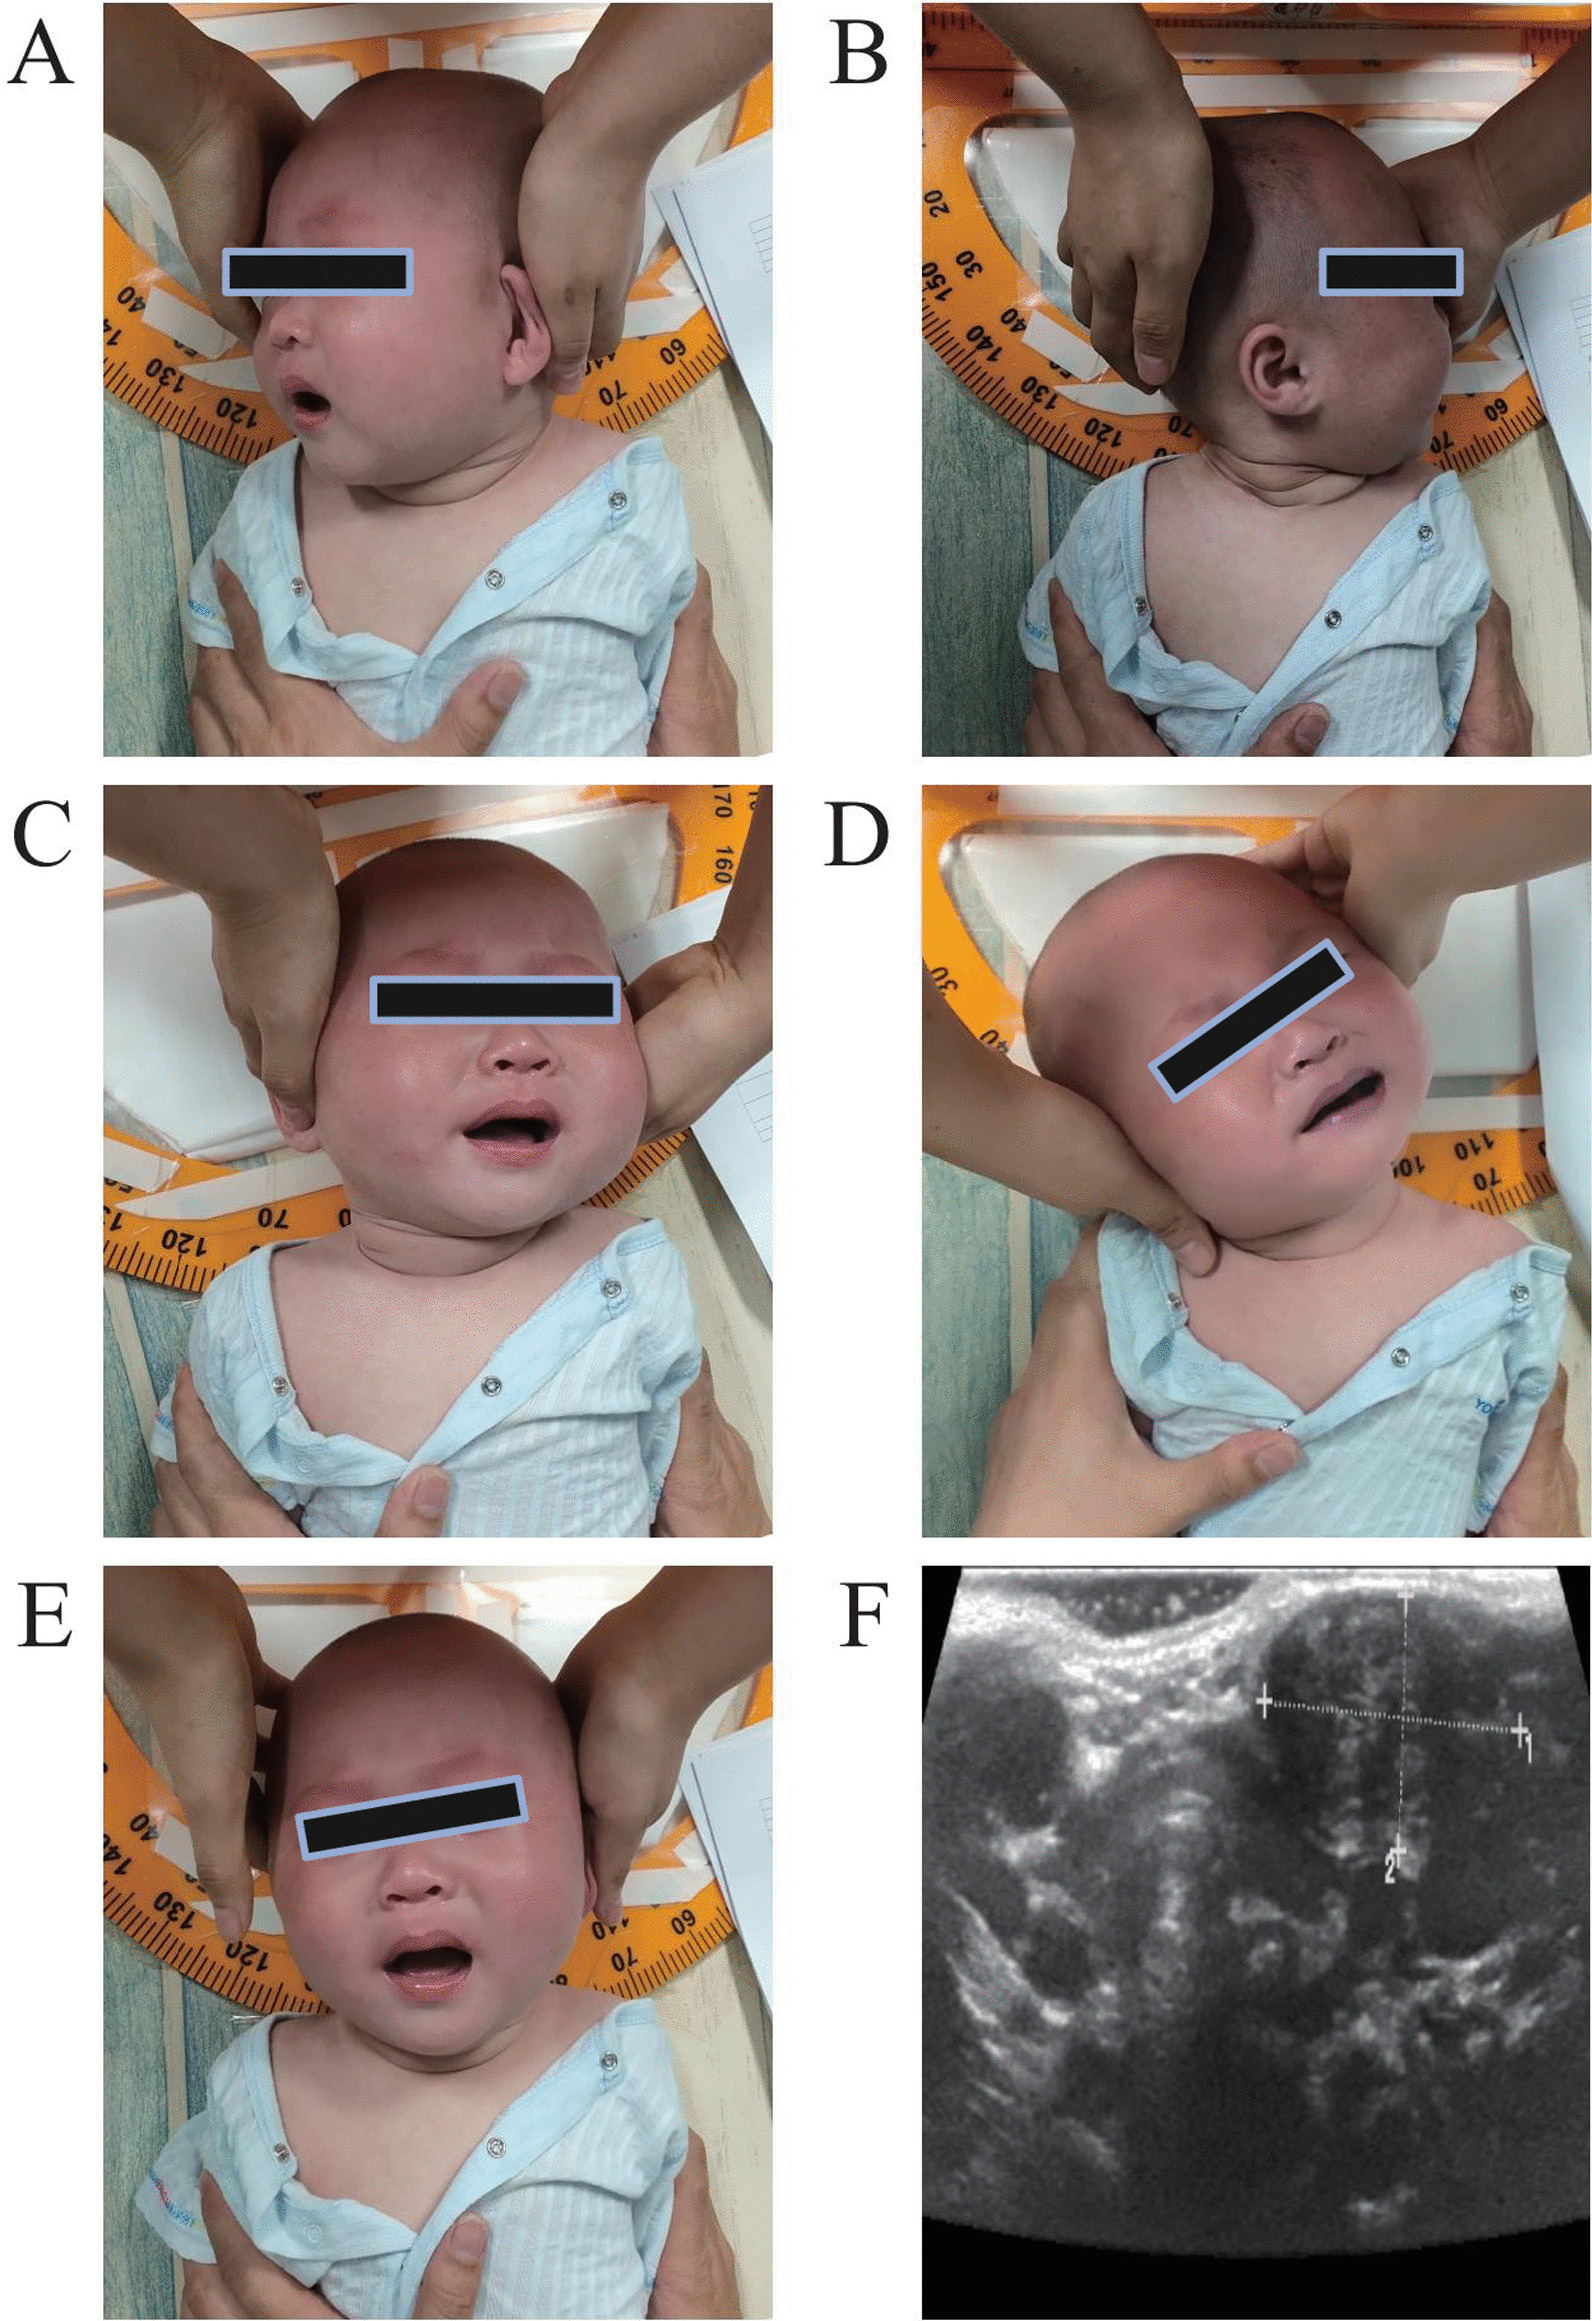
A patient with right congenital muscular torticollis. The patient has limited head rotation to the right but not to the left (a, b). There is limited head flexion to the left side but not to the right side (c, d). The patient’s head is tilted to the right under normal conditions (e). Ultrasound shows significantly thicker sternocleidomastoid muscle on the affected side than on the healthy side (f)
